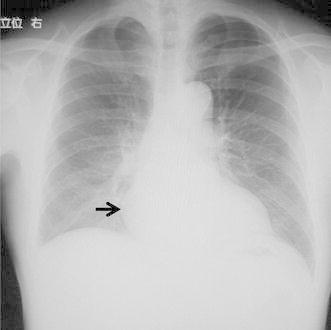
Chest radiography shows a mass on the right lower mediastinum
